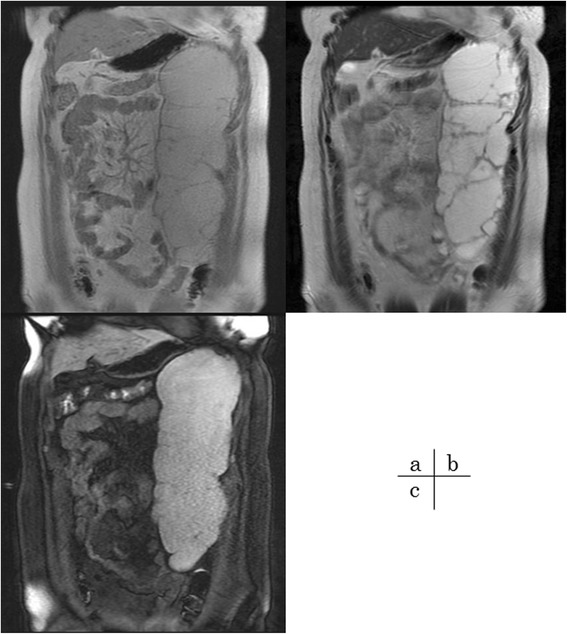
A hyperintense multicystic tumor on magnetic resonance imaging (MRI). a T1-weighted imaging. b T2-weighted imaging. c Fat-suppression T1-diffusion-weighted imaging. The tumor contains blood component and no fatty component
